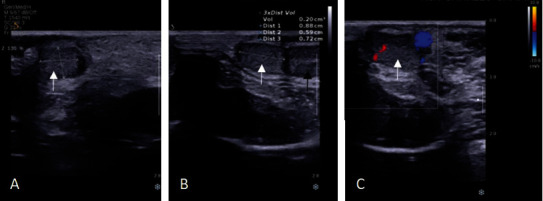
Ultrasonography in a seven-year-old boy showing a slightly echoic oval-shaped structure (white arrow), compared to the left testicular parenchyma (black arrow), located between the left epididymis and the left testicle, and measuring 0.9 × 0.6 × 0.7 cm. It is completely separated from the left testicle and shows mild intralesional Doppler flow (C) with a slight posterior reinforcement, compatible with a supernumerary testis.
